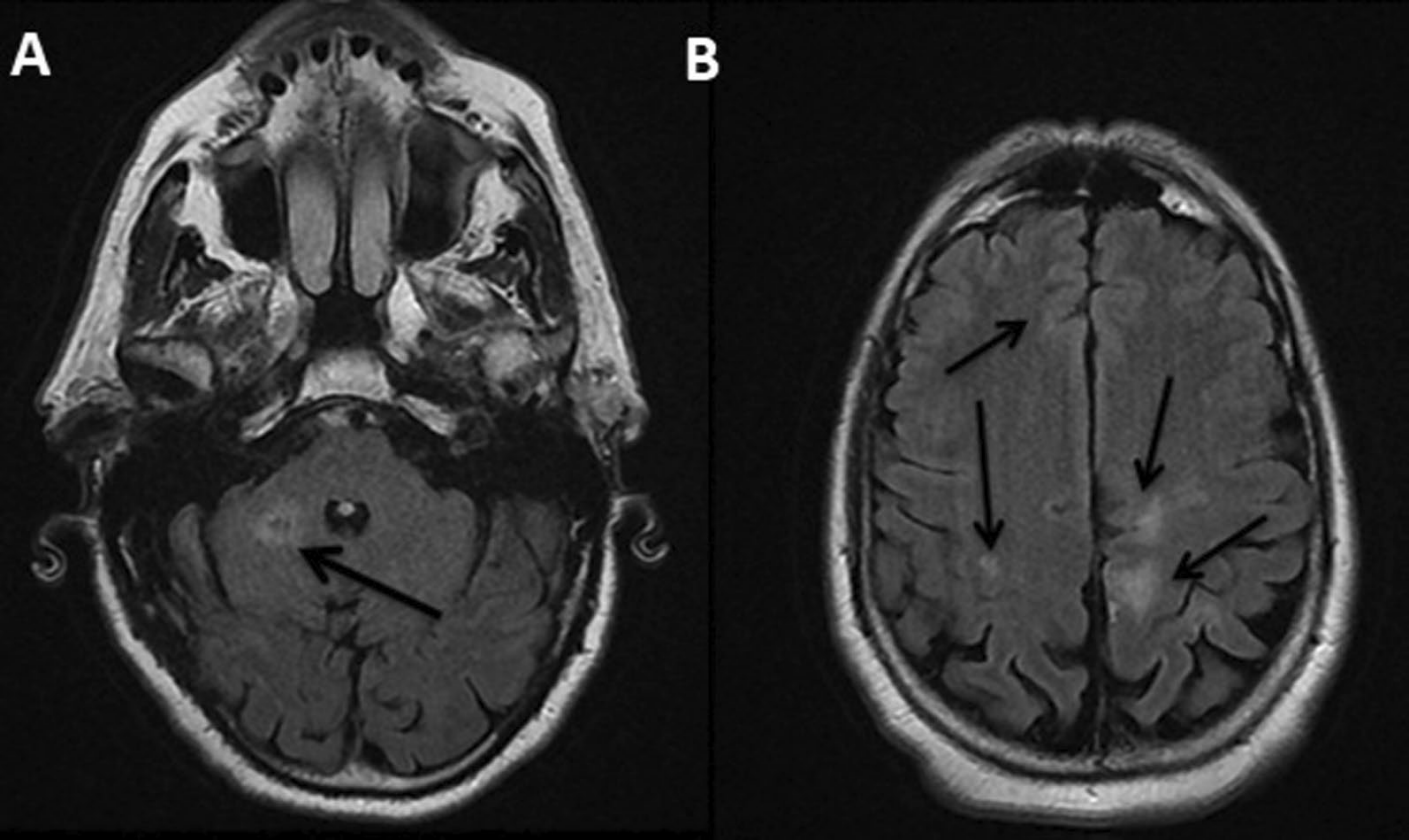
A + B Axial views of brain MRI showing frontal, occipital and cerebellar white matter signal abnormalities
